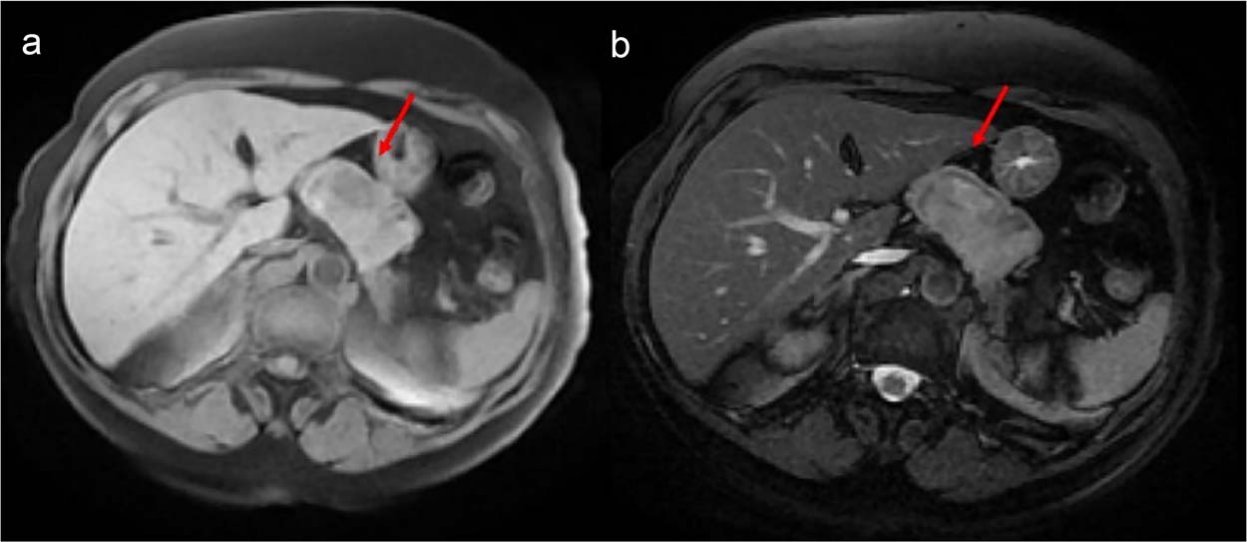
Abdominal MRI showing a heterogeneous intermediate T1 signal mass (a) with slight hyperintensity on T2-weighted images (b), without fatty components, closely contacting the stomach but distant from the liver.
